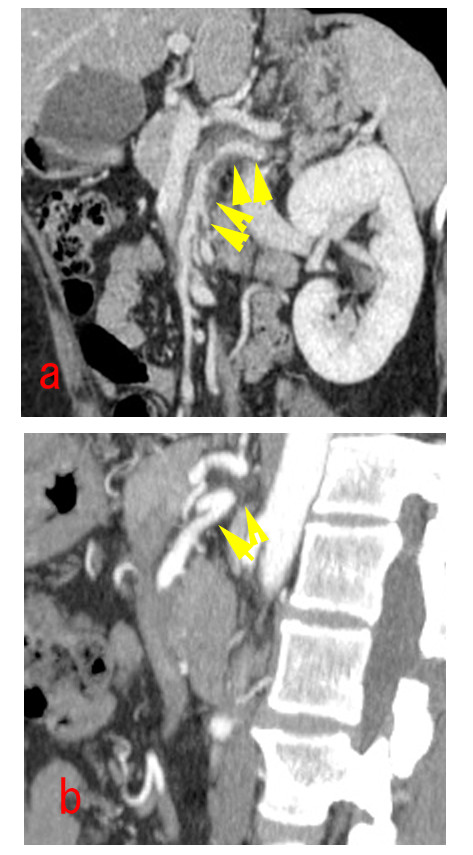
Sakamoto's type III dissection of the SMA. (a) preoperative MPR image of an abdominal enhanced CT scan show isolated dissection of the SMA began just after the orifice of the SMA and extended to the distal portion, with ULP and the narrow true lumen being compressed by the thrombosed false lumen. (b) postoperative 3 year abdominal enhanced CT scan show a thrombosed false lumen completely resolved without progressive dilation of ULP.
